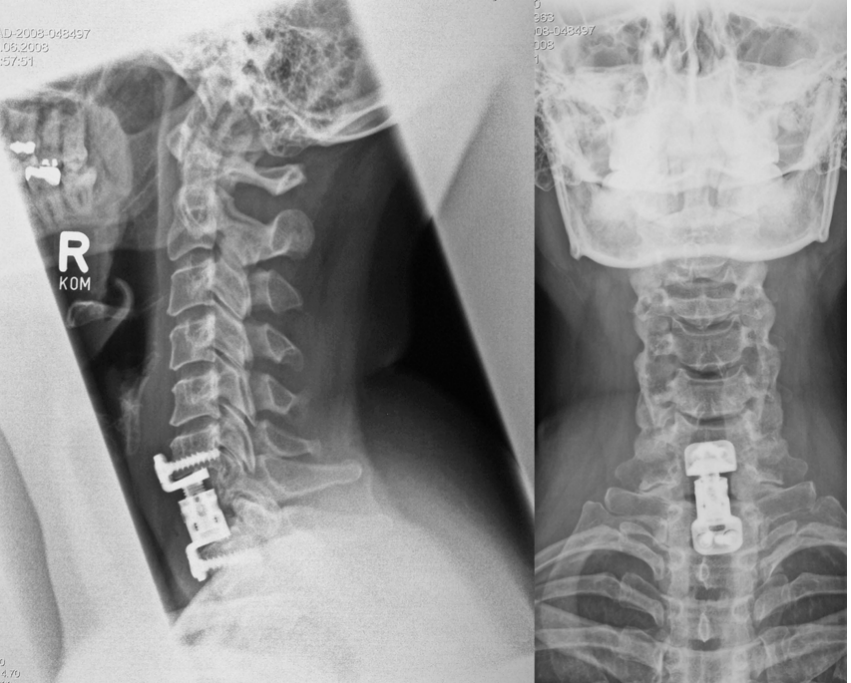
Radiography of the cervical spine in two levels. Six months after corporectomy of C7 and cage implantation which show that the correct position of the cage has been maintained and the physiological alignment of the cervical spine is affirmed.
